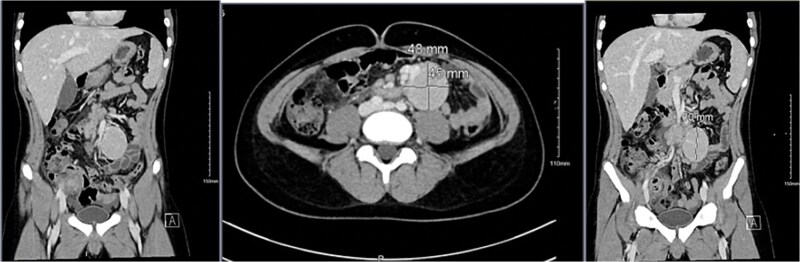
A CT scan of the abdomen revealed an appendicular abscess measuring ⁓40 mL with an associated appendicolith and a soft tissue mesenteric mass measuring 6 × 4 cm, accompanied by enlarged mesenteric lymph nodes.
